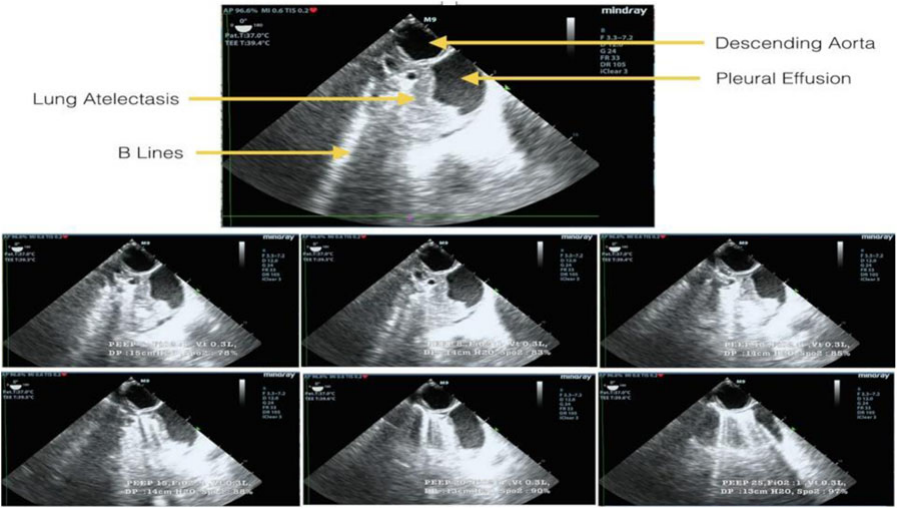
Transesophageal echocardiography (TEE) showing a posterior basal lung (PBL) atelectasis and disappearance of lung consolidation (hepartization or tissue-like appearance) .A formation of B-lines during lung recruitment maneuver (using incremental PEEP method), indicating a part of recruited lung
